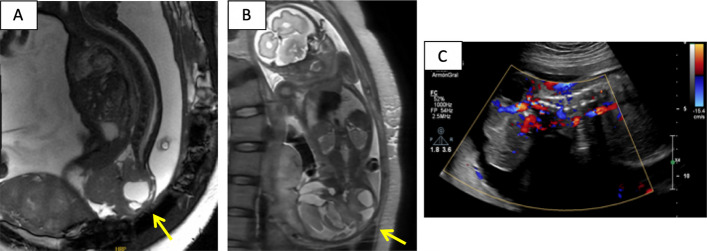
Fetal MRI (A and B) and transabdominal ultrasound Doppler study (C) at week 29 + 6. Solid-cystic sacrococcygeal tumor corresponding to a teratoma (type 2 of the Altman classification) (arrows in A and B), highly vascularized in the Doppler study (C)
